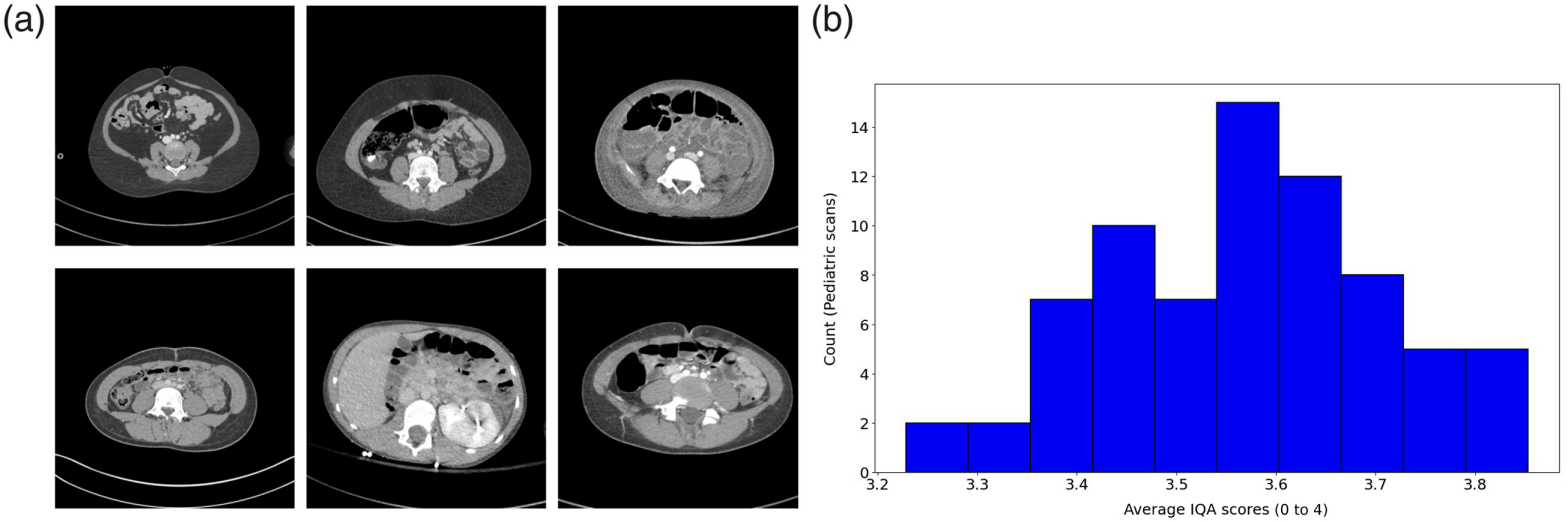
(a) Sample CT images in the pediatric scan dataset (window width: 500; center: 1050). (b) Model predicted IQA scores demonstrate good agreement with the clinical diagnostic quality (IQA score>3) for clinical pediatric abdomen CT images.
